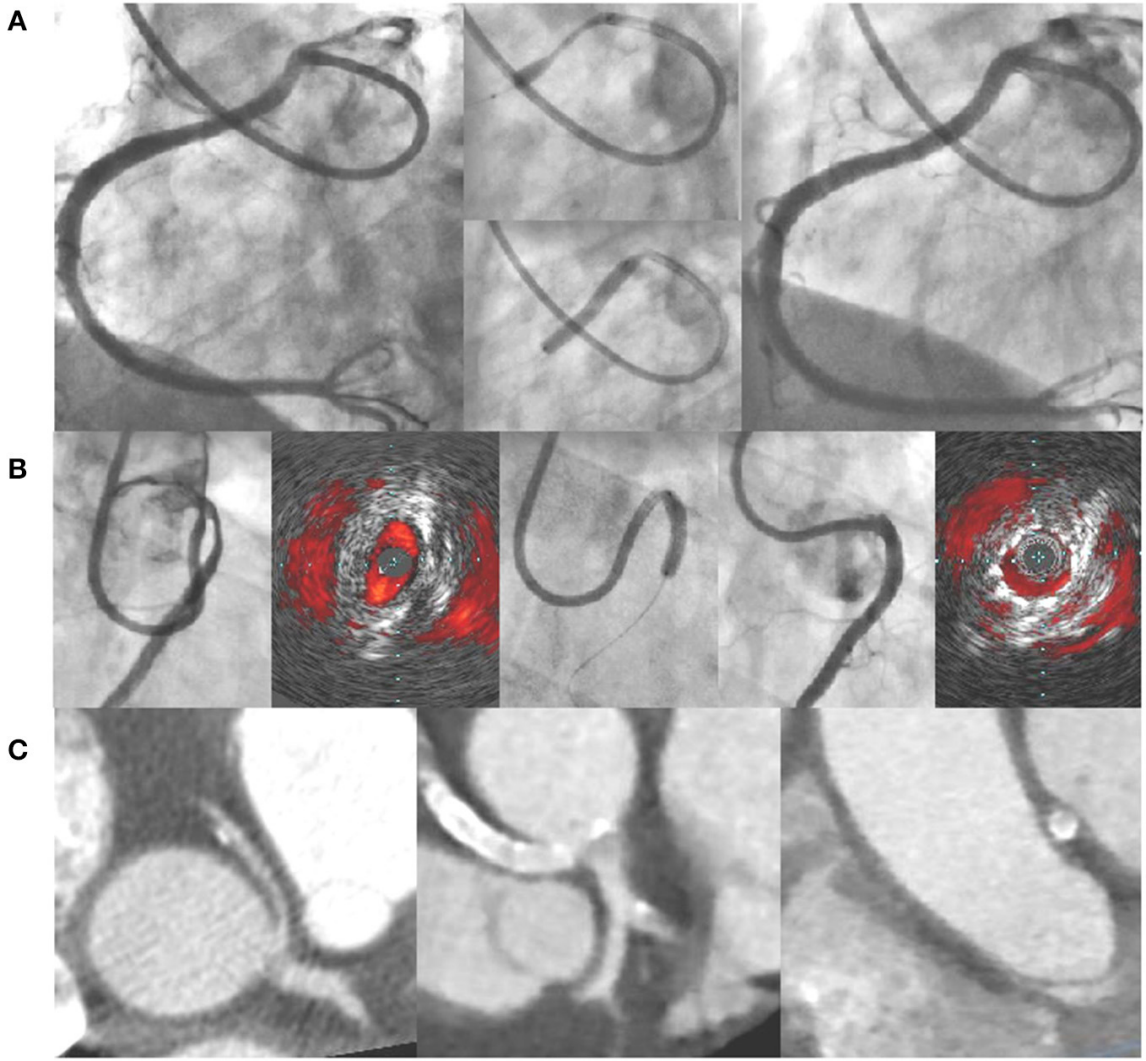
(A) Example of angioplasty with stenting of a right AAOCA (pre-PCI, stent deployment, post-PCI); (B) angioplasty with stenting under IVUS guidance of a right AAOCA associated with an intramural pathway (pre-PCI, stent deployment, post-PCI); (C) CCTA images of a right AAOCA treated by angioplasty with stenting (pre-PCI and post-PCI at-6 month). AAOCA, anomalous aortic origin of a coronary artery; CCTA, coronary computed tomography angiography; PCI, percutaneous coronary intervention; IVUS, intravascular ultrasound.
